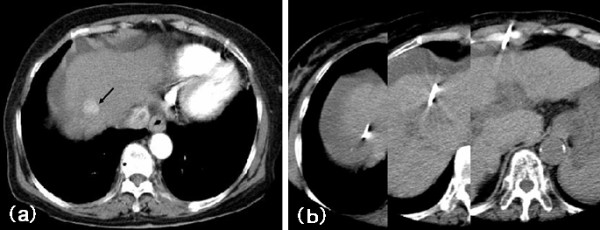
A 76-year-old woman. Arterial-phase CT shows cirrhotic liver with a highly enhanced HCC in the right lobe (arrow) and a small amount of ascites (Fig. 3a). The needle entry site was decided to avoid through acites and right lung. GNW was accessed to HCC (Fig. 3b). The lesion was located 11 cm from the needle entry site.
